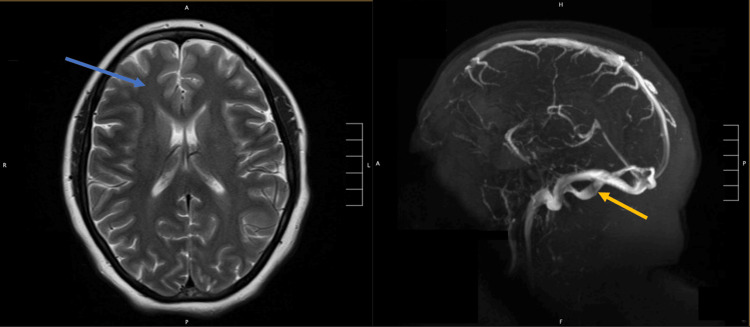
T2-Weighted MRI (left) revealed a subacute frontal infarct (blue arrow) and MRV (right) revealed a cerebellar lacunar infarct (orange arrow); both findings confirmed that the patient had a stroke. MRI: Magnetic resonance imaging; MRV: Magnetic resonance venography
